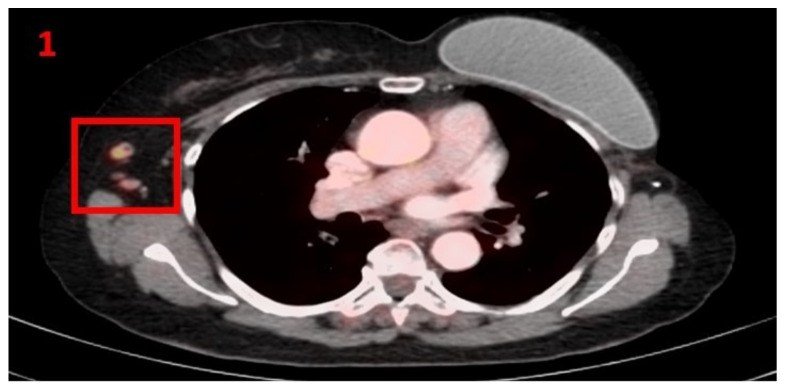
[18F]FDG PET-CT showing hypermetabolic uptake in the 1st patient with lymphadenopathy in the right axillary region (red square).
